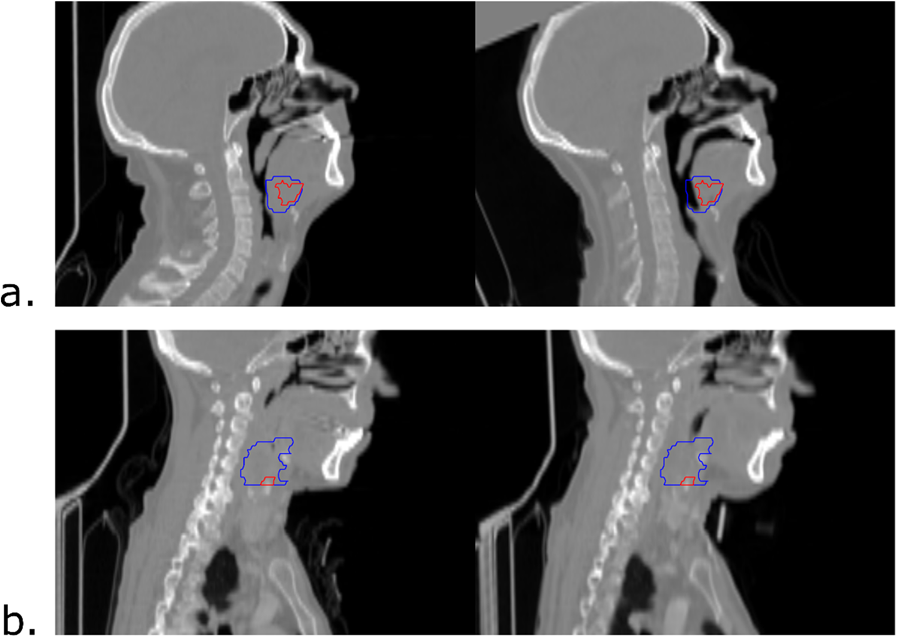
Examples of both inaccurate and accurate structures matching. Left: pre-treatment CT. Right: post-treatment CT. a Inaccurate structures matching. Although the skull, the jaw, and the top of the spinal cord are aligned, the tongue of the patient appears in a different position between the two scans, leading to a misalignment of pertinent structures for tumor recurrence analysis. b Accurate structures matching. The skull, jaw and spinal cord are aligned and no anatomical differences between the soft tissues were found nearby the tumor. Blue: pre-treatment tumor. Red: post-treatment tumor
